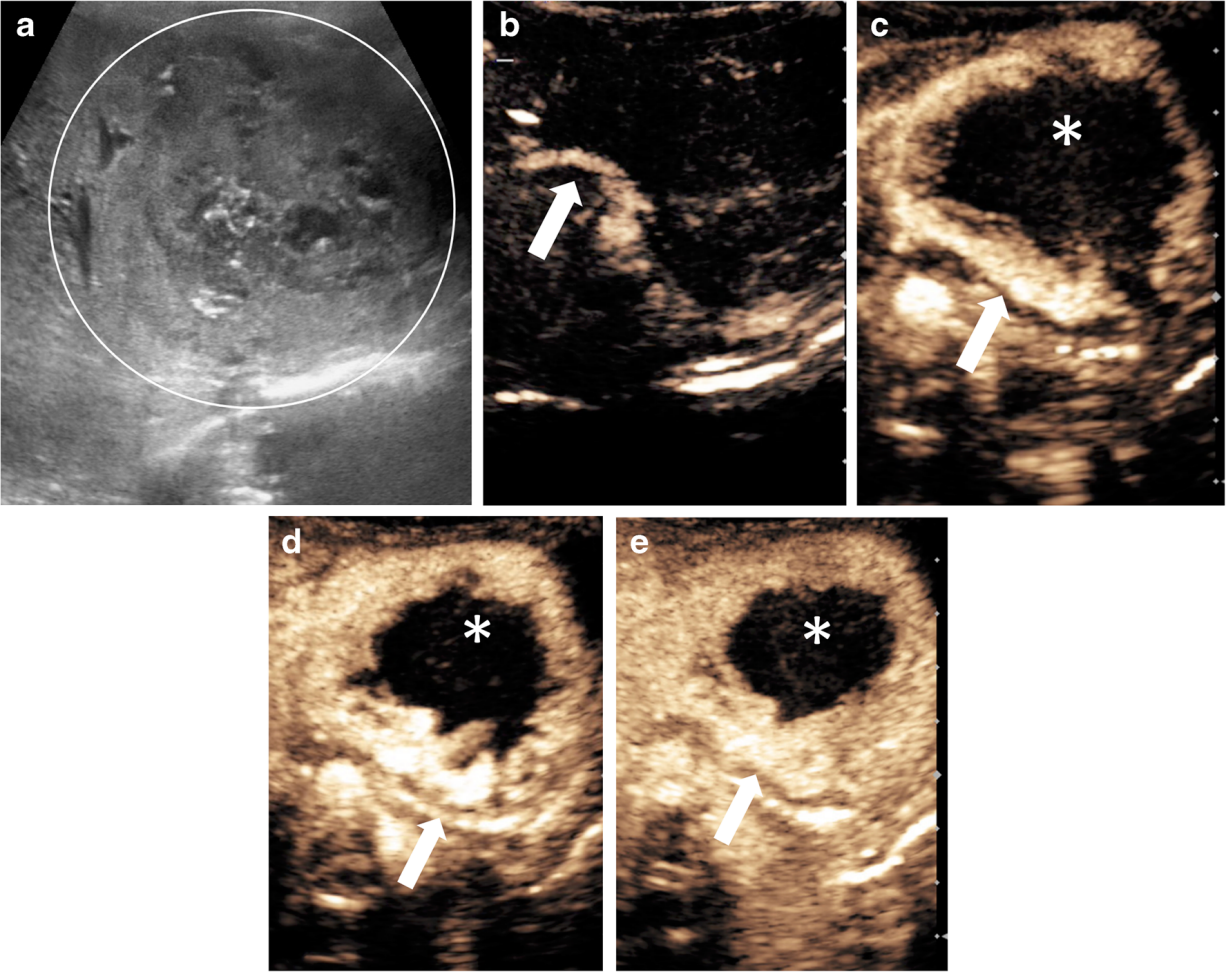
Rapidly involuting congenital hemangioma in a 3-week-old boy with a congenital mass of the left liver lobe. a Transverse gray-scale US shows a large heterogeneous lesion (*circle*) within the left liver lobe. The volume of the lesion at initial scan was 41 mL. b–e Transverse contrast-enhanced ultrasound (CEUS) of the liver in contrast-only mode in the arterial phase at 11 s (b), 14 s (c) and 18 s (d), and in the portal venous phase 60 s after injection of the contrast agent (e). Rapid nodular enhancement progresses from the periphery (*arrows*) to the center, with incomplete enhancement in the central aspect (*asterisks*) of the lesion. In the follow-up US scans (not shown) except for residual calcifications there was near-complete regression of the lesion. Imaging features are typical for rapidly involuting congenital hemangioma. In this child, at 9 months of age the volume of the lesion had decreased at 2.6 mL, and at 5 years of age the lesion was barely visible with a measured residual volume of 0.4 mL
